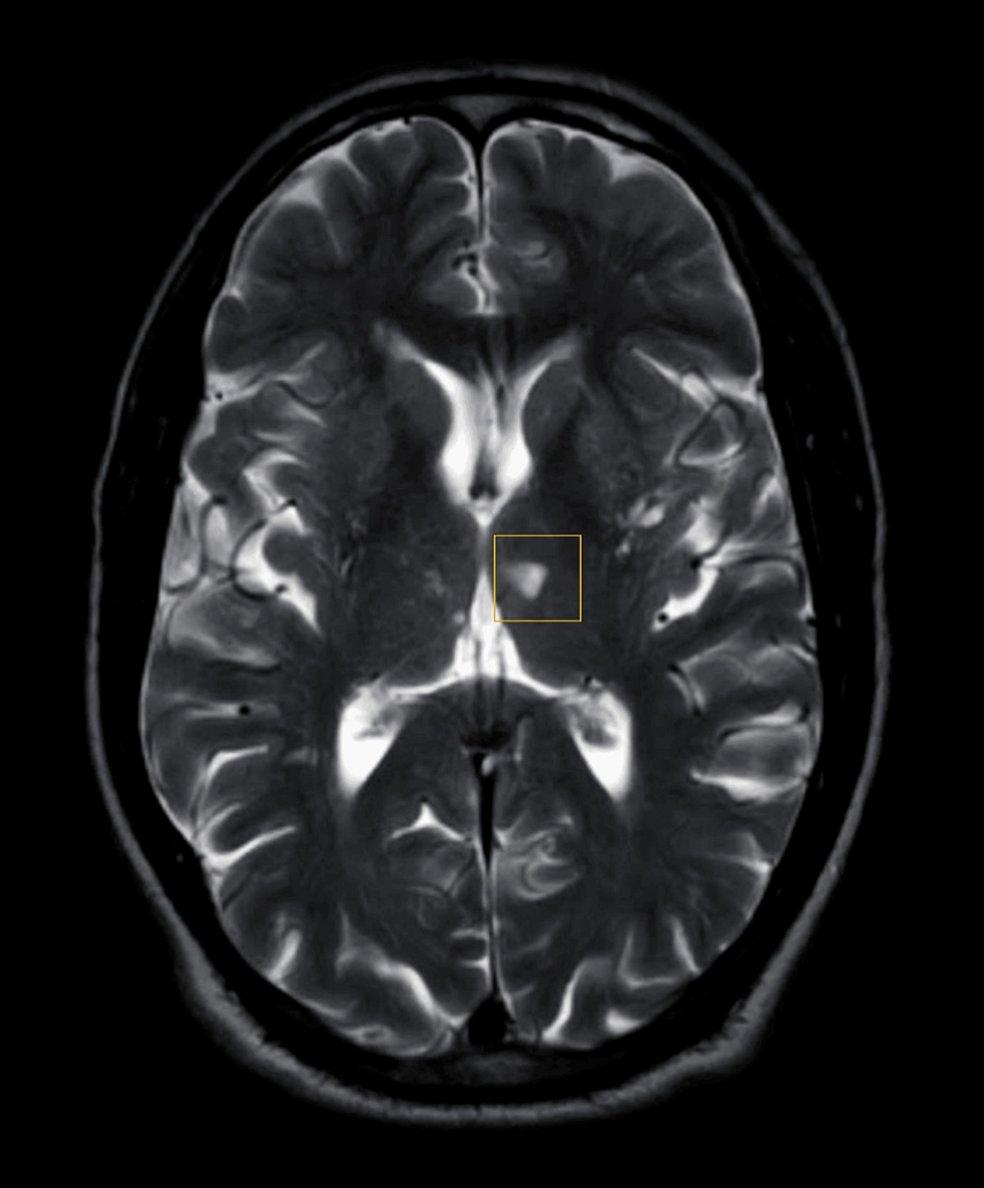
T2-weighted MRI scan showing left thalamic infarct The highlighted area of focus shows the thalamic infarct. MRI: magnetic resonance imaging
